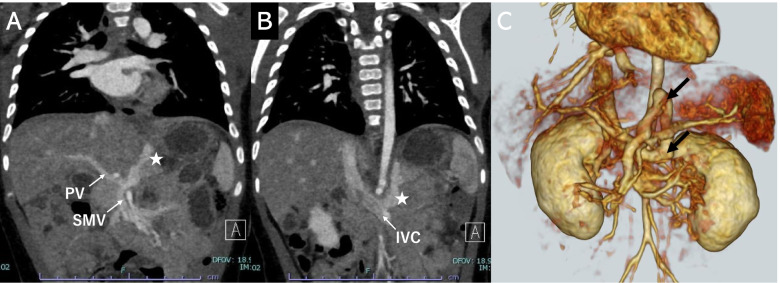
Abdominal contrast-enhanced computed tomography (CT). A The CPSS branches from the splenic vein and rises once cephalad. B The CPSS arches and descends at a level close to the stomach and flows into the left renal vein. C Detailed morphology of the CPSS (arrow) on three-dimensional CT. CPSS, congenital portosystemic venous shunt; PV, portal vein; SMV, superior mesenteric vein; IVC, inferior vena cava
